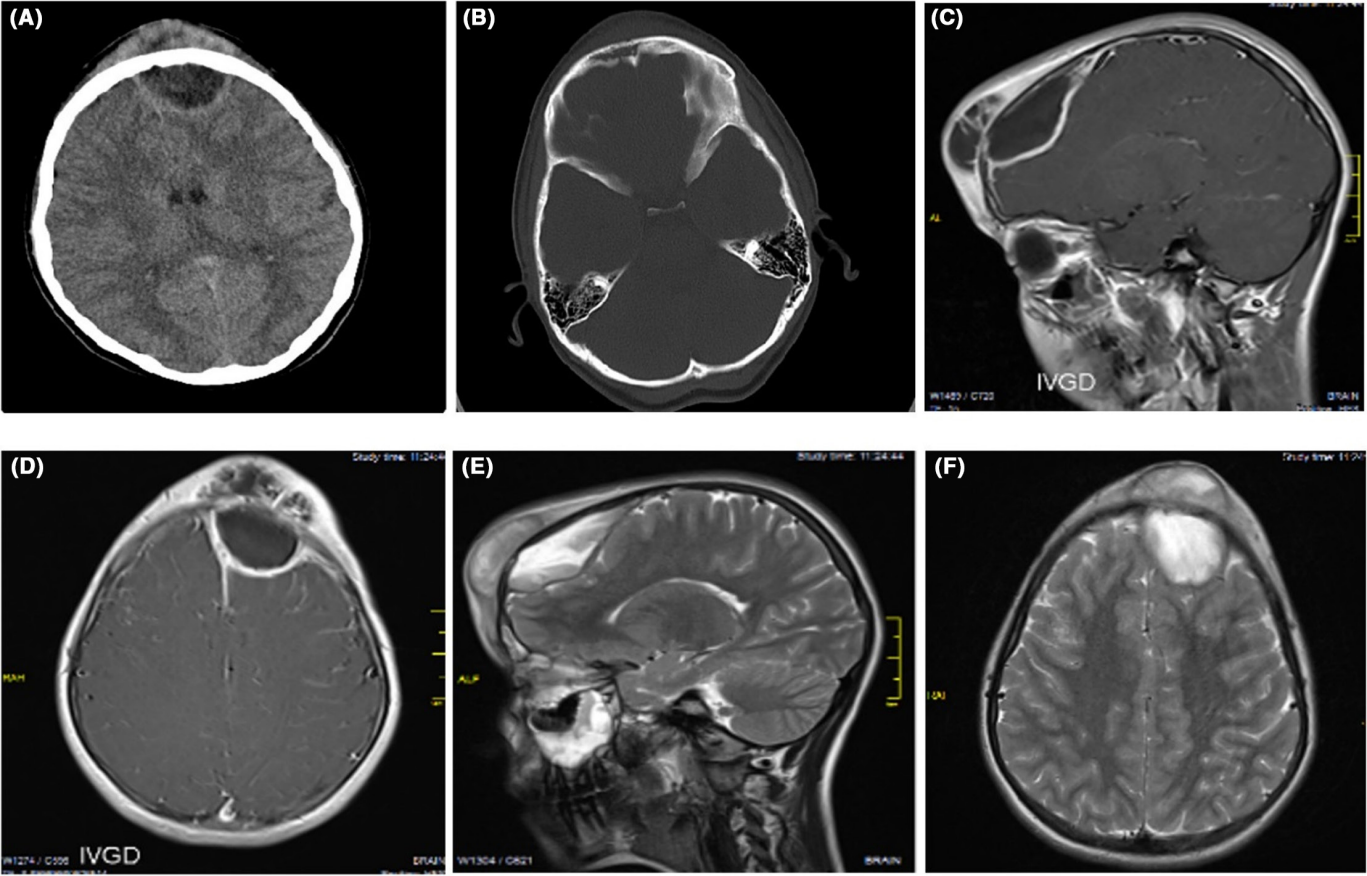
CT scan and MRI imaging of the patient. (A) Axial brain CT scan showing swelling of the overlying scalp and a hypodense frontal lobe abscess with a hyperdense rim. (B) Axial brain CT scan indicating erosion of the left frontal bone and opacification of the frontal sinus. (C and D) Contrast enhanced T1‐weighted sagittal and axial brain MRI sections, respectively, showing Pott's puffy tumor and intracranial extradural abscesses. (E and F) T2‐weighted sagittal and axial brain MRI sections showing a subperiosteal abscess in the forehead and an epidural abscess.
